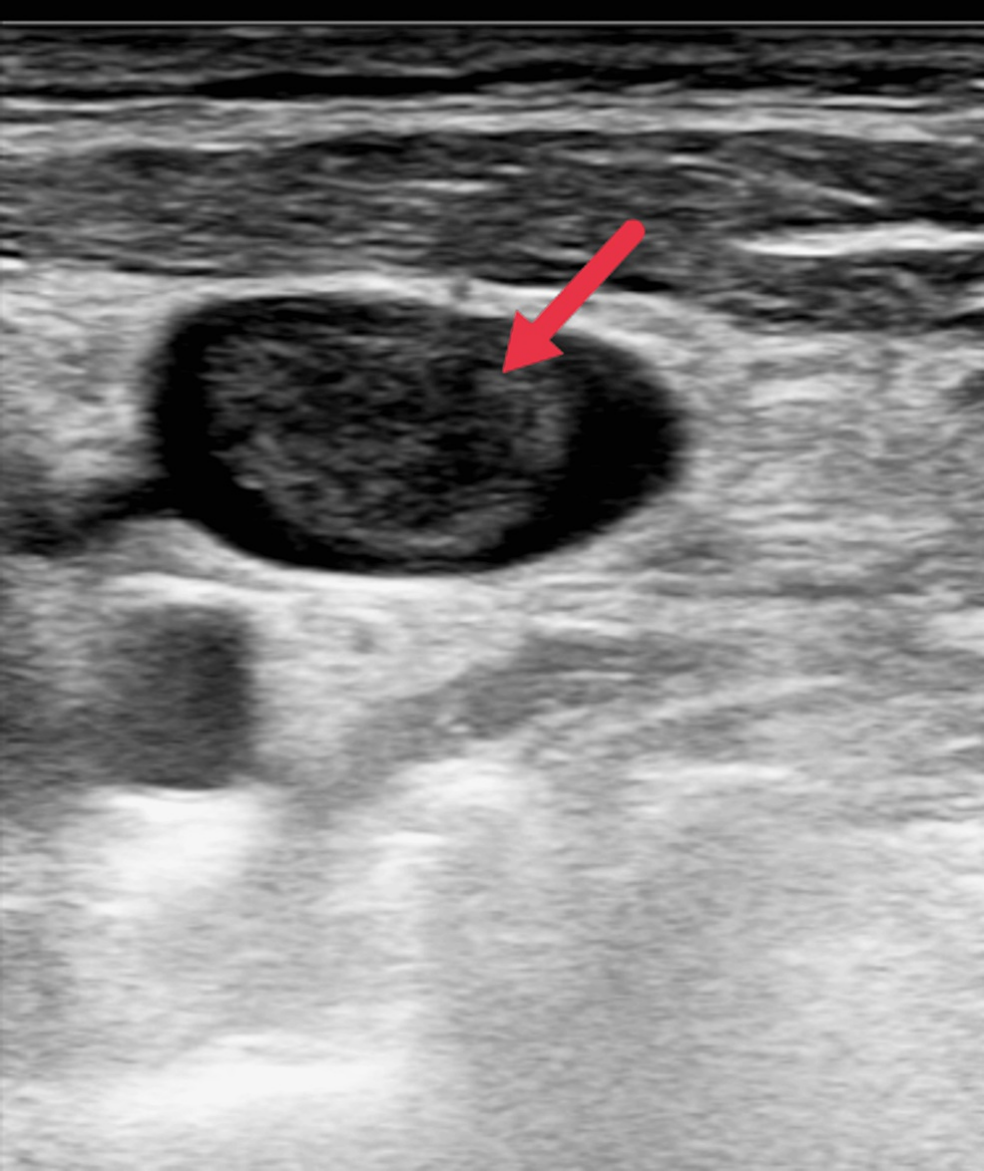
Point-of-care ultrasonography of left internal jugular vein showing echogenic thrombus (red arrow).
